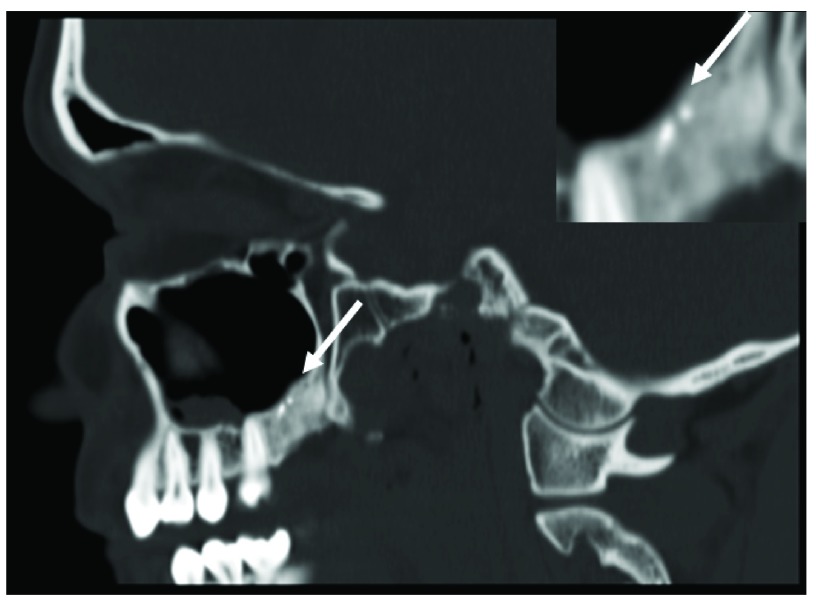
Sagittal section of non-contrasted CT scan shows two retained remnants of gutta-percha (white arrow) in the left maxilla in the location of previous dental extractions. Inset in the top-right corner reveals a zoomed image of the two gutta-percha remnants.
